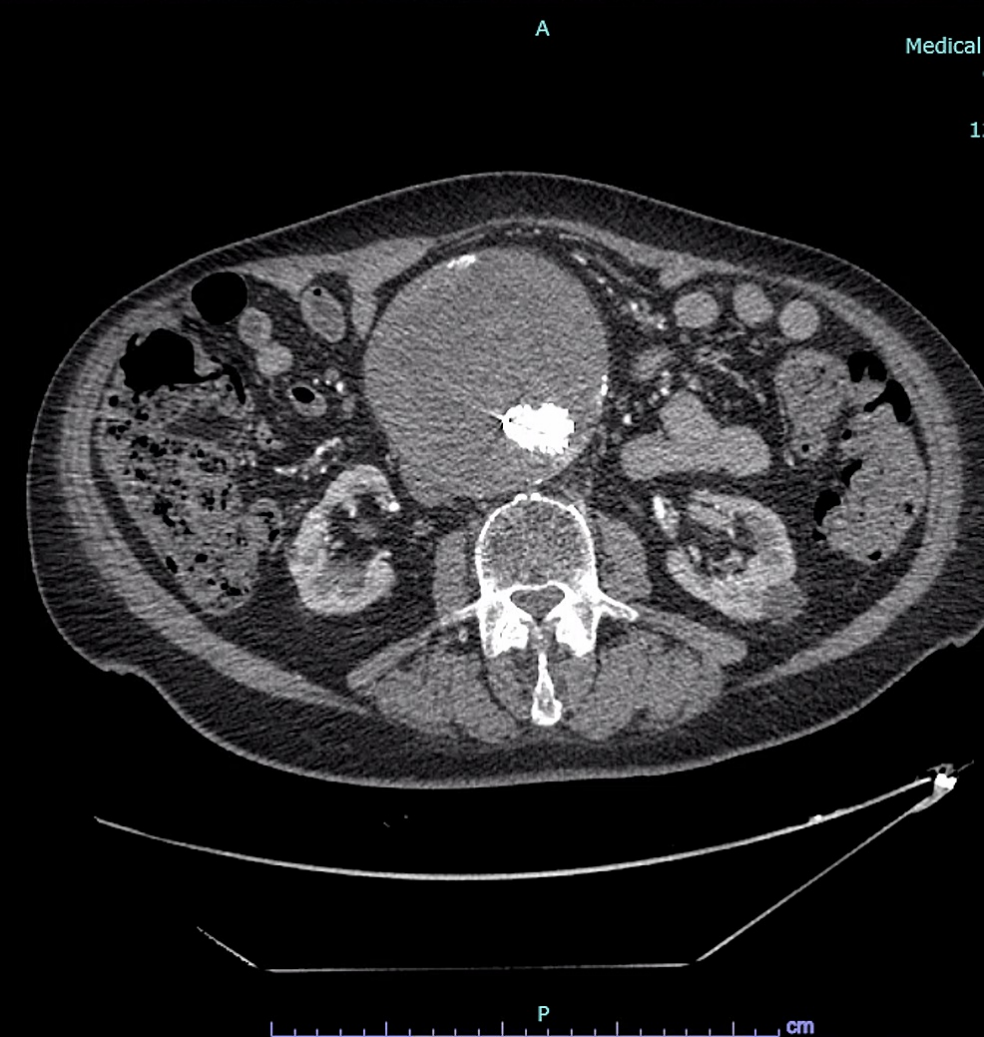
Computed tomography angiography of the abdomen and pelvis revealing large abdominal aortic aneurysm with endograft in place
